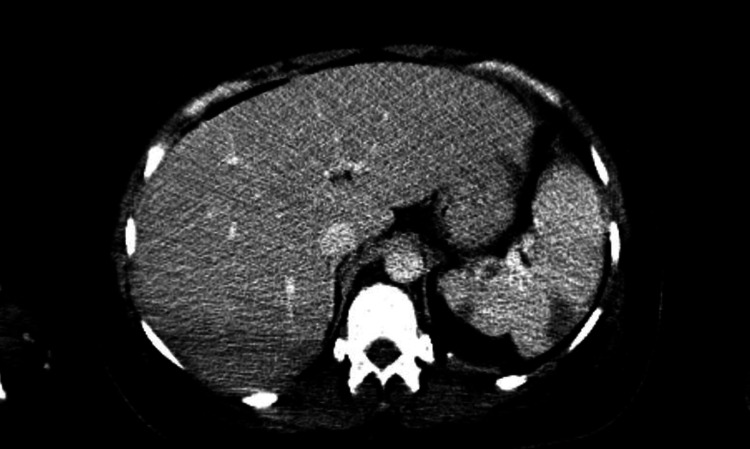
CT of the abdomen showing splenic infarcts.
